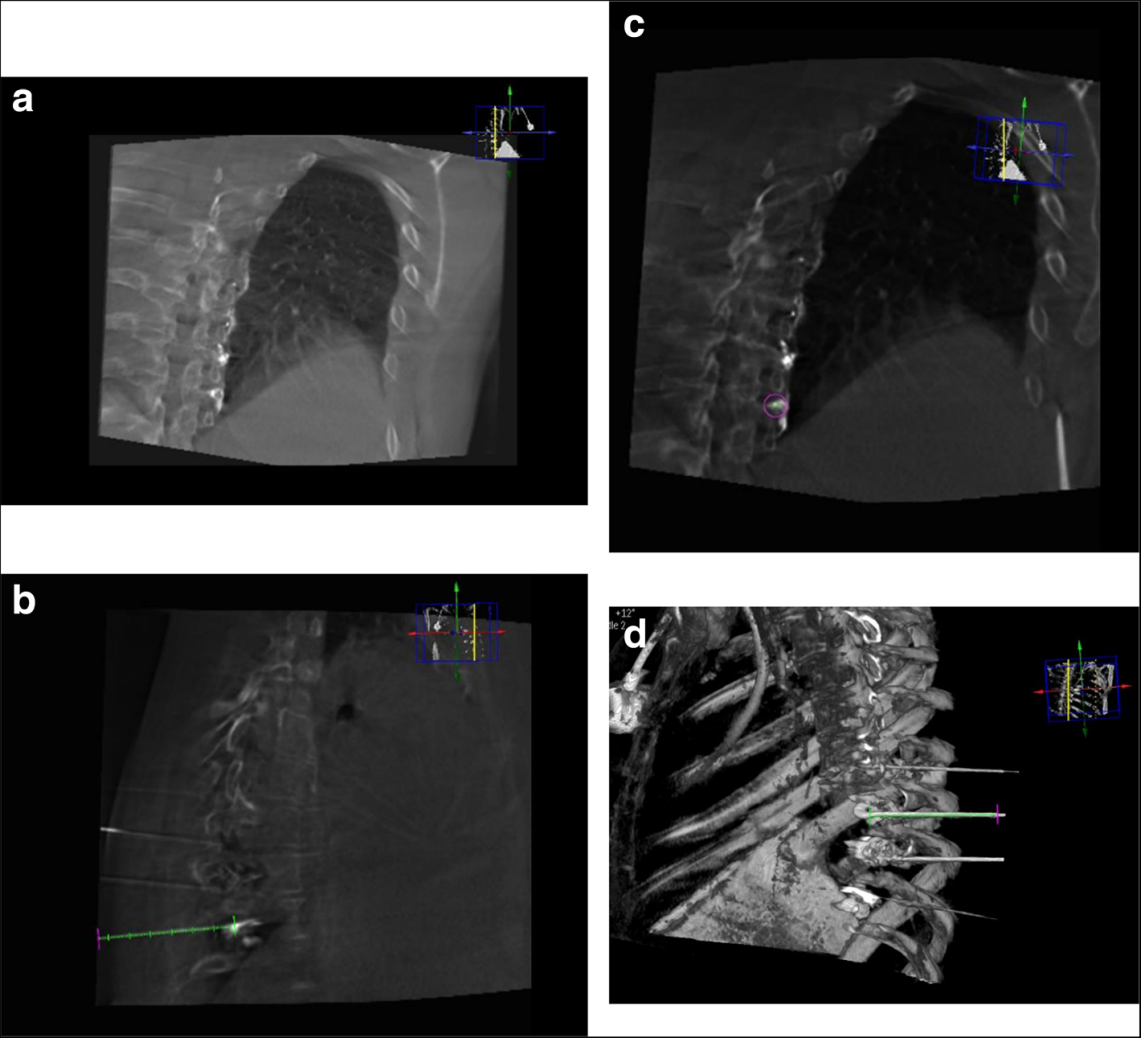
a, b, c and d: image sequence describing the Xper CT-Fluroscopy technique of thoracic transforaminal approach.
